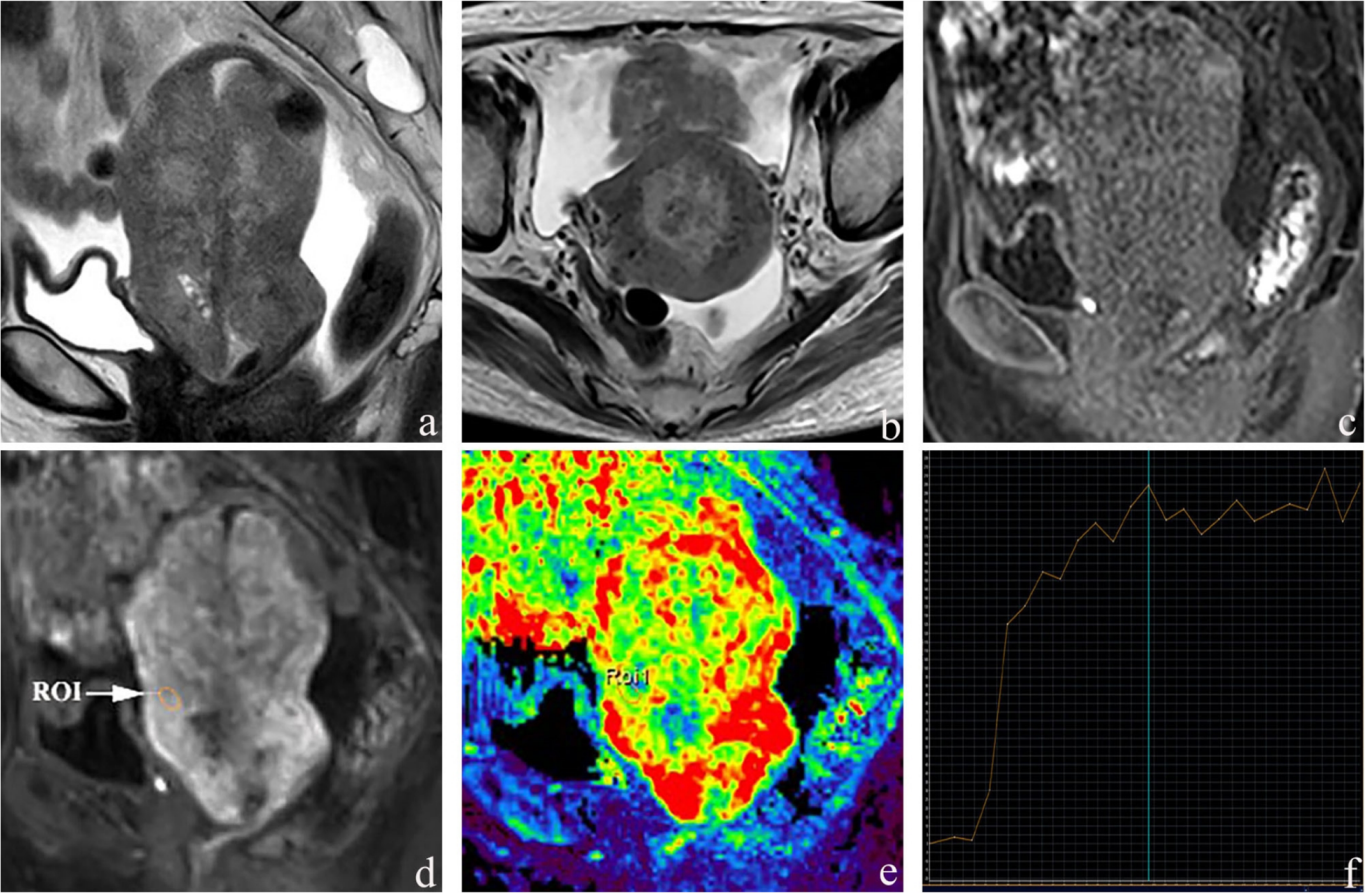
MRI images. a Sagittal T2-weighted image shows that the uterus was replaced by diffuse inhomogeneous signal intensity. b Axial T2-weighted image shows that the lesion is ill defined and diffusely invades the myometrium. c, d Sagittal T1-weighted image shows the lesion appeared with hypointensity and inhomogeneous enhancement. e Colour perfusion map shows the slightly lower vascularity of the tumour (green area), while the greater vascularity is depicted in red (the normal myometrium). f The dynamic enhanced curve of the USC: avid enhancement, with both early and persistent enhancement
